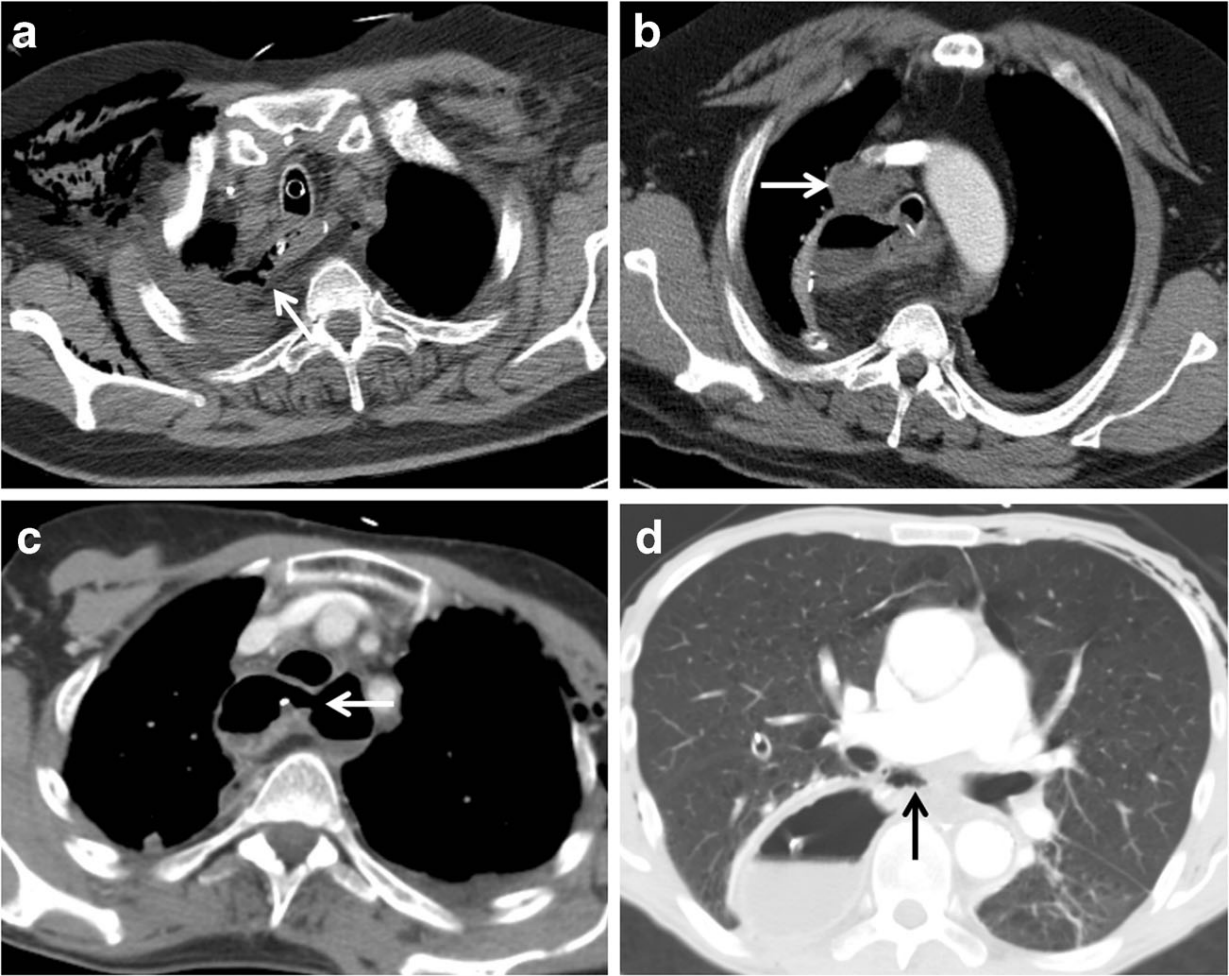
Examples of CT findings associated with the presence of anastomotic leakage after esophagectomy. A: Image shows a fistula between the gastric tube and right pleural cavity (*arrow*). B: Image shows a fluid collection (*arrow*) in the mediastinum. C: Image shows a visible discontinuity of the esophagogastric wall (*arrow*). D: Image shows a mediastinal air cavity (*arrow*) after esophagectomy
